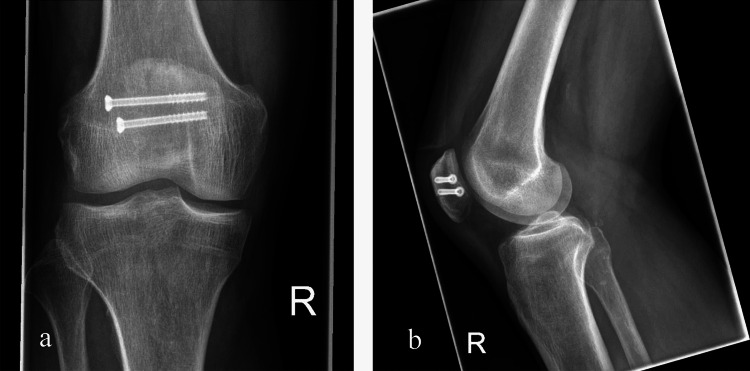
Anteroposterior (a) and lateral (b) X-ray views of a simple transverse patellar fracture managed operatively using screw fixation Reproduced from Pesch et al. [28], licensed under Creative Commons Attribution 4.0 International License (CC BY 4.0).
